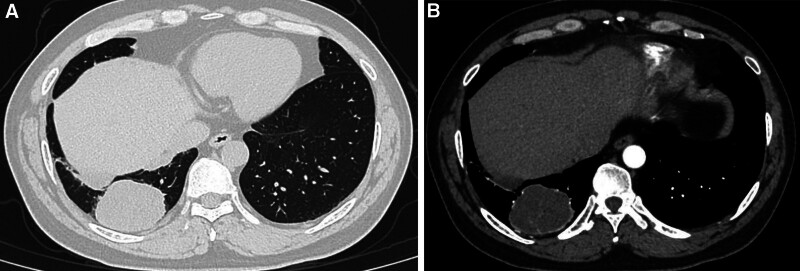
(A) CT plain scan reveals multiple clustered soft tissue density shadows in both lungs. (B) CT enhancement suggests mild to moderate continuous enhancement of the mass.
